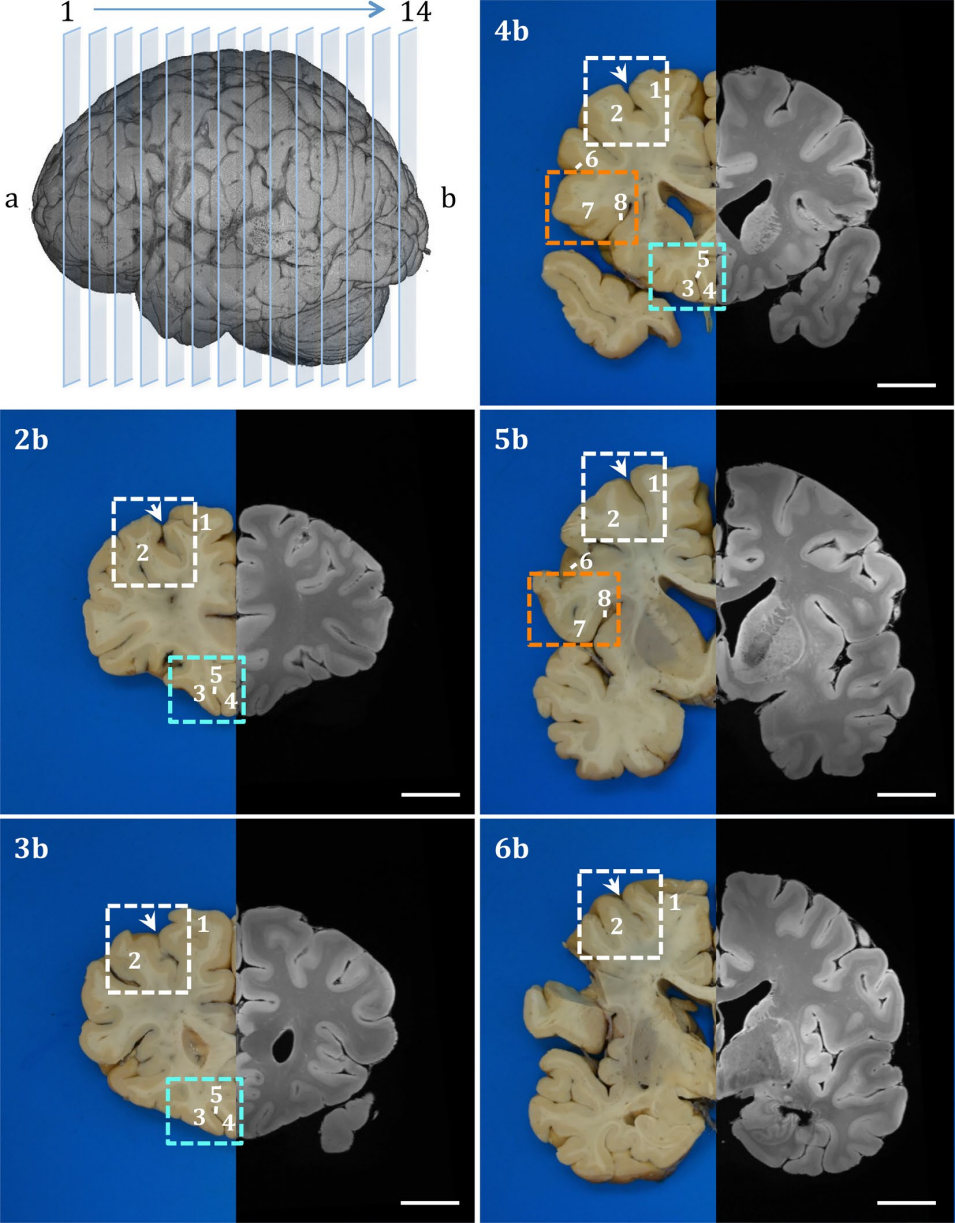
Systematic sampling strategy for the cortical regions of the frontal lobe. The 3-D MRI image on top left-hand corner represents slicing of the brain on coronal plane. Each slice numbered consecutively in rostral-caudal axis and labelled as anterior (a) or posterior (b) when digitally photographed. Images 2b–6b show gross brain coronal slices (posterior plane) with mirrored structural MR image from the left hemisphere. Key neuroanatomical regions middle frontal gyrus (white rectangle), orbitofrontal cortex (orbital gyri and gyrus rectus: turquoise rectangle) and inferior frontal gyrus (orange rectangle) were sampled in serial coronal slices systematically with their accompanying subcortical white matter. Major sulci were used as landmarks for region and anatomical boundary identification. Annotations—1: superior frontal gyrus, 2: middle frontal gyrus, 3: medial orbital gyrus, 4: gyrus rectus, 5: olfactory sulcus, 6: inferior frontal sulcus, 7: inferior frontal gyrus, 8: circular insular sulcus, arrow: superior frontal sulcus. Scale bar = 2 cm
